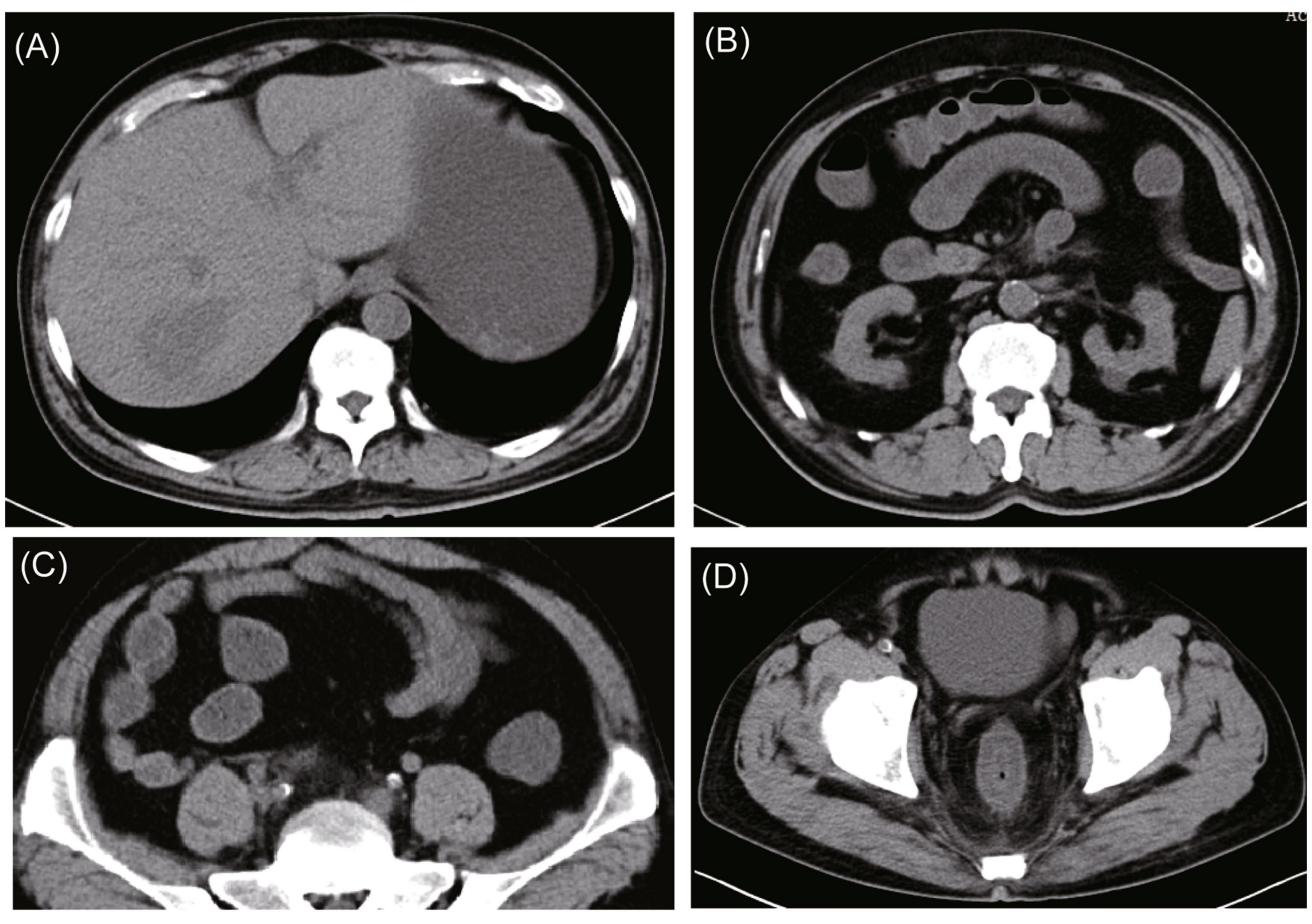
Abdomen and pelvic CT scan on hospital day 1. (A) The liver shows a smooth contour with well-defined margins and proportionate lobar anatomy. A patchy hypodense lesion is observed in the posterior segment of the right hepatic lobe, measuring approximately 5.9 × 4.8 cm, with ill-defined borders. (B,C) The bowel loops demonstrate marked gaseous and fluid distension, consistent with impaired motility. (D) The rectal wall appears thickened, with increased density of the surrounding mesenteric fat, showing stranding and streak-like opacities suggestive of inflammatory changes. No significantly enlarged lymph nodes are identified in the abdominal cavity, retroperitoneum, pelvic walls, or bilateral inguinal regions.
